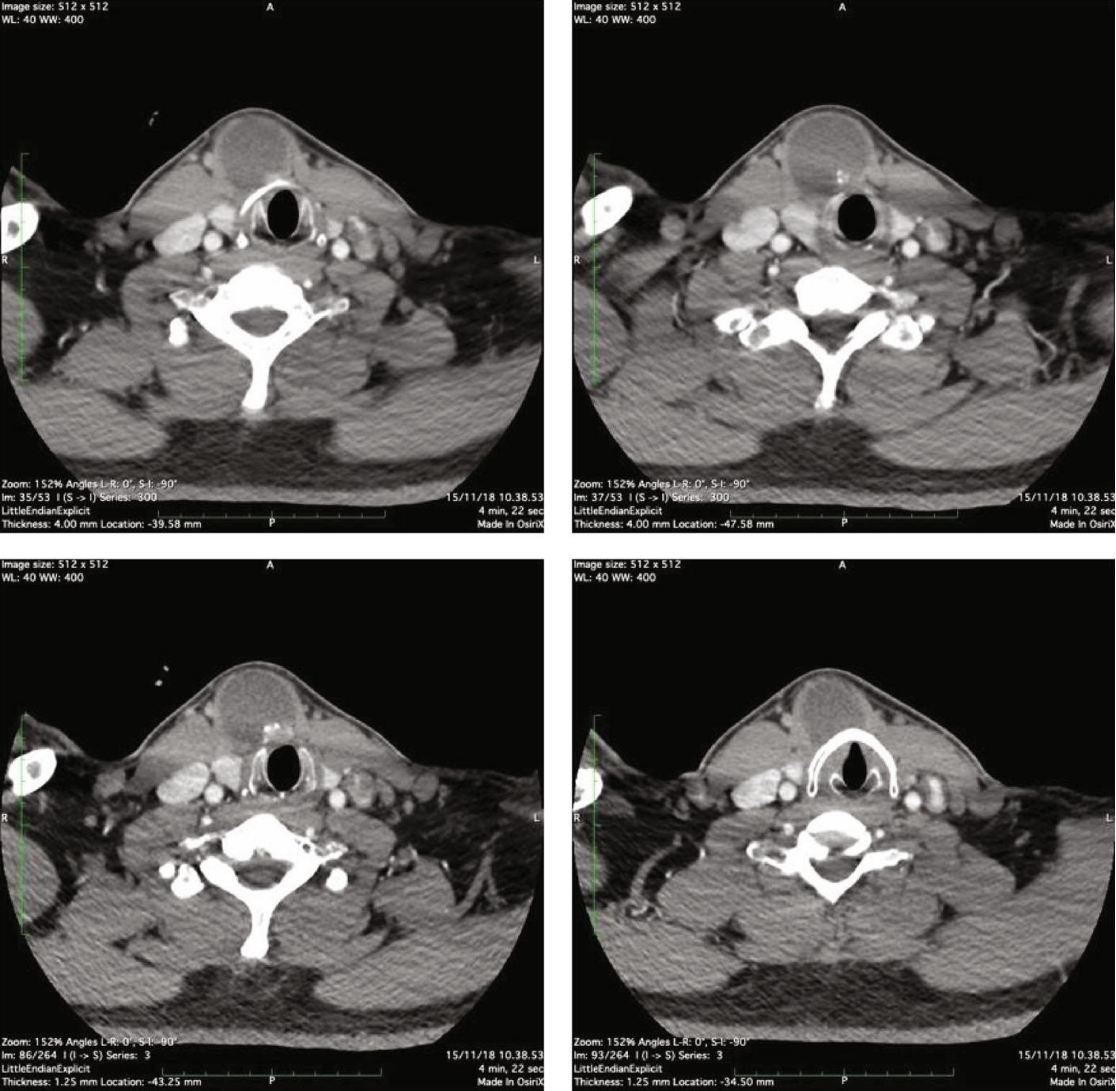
Cervical axial CT scan with contrast. Complex cystic mass in the right parasagittal anterior colli area, appears to bulge to the level of the larynx.
